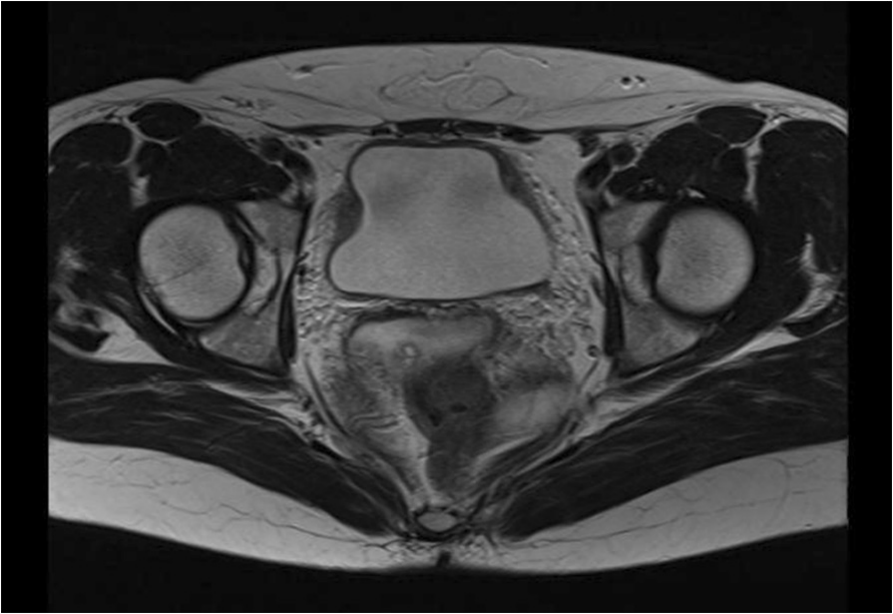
Magnetic resonance imaging after the bladder capacity improved to 75mL showed a well-rounded muscle layer.
